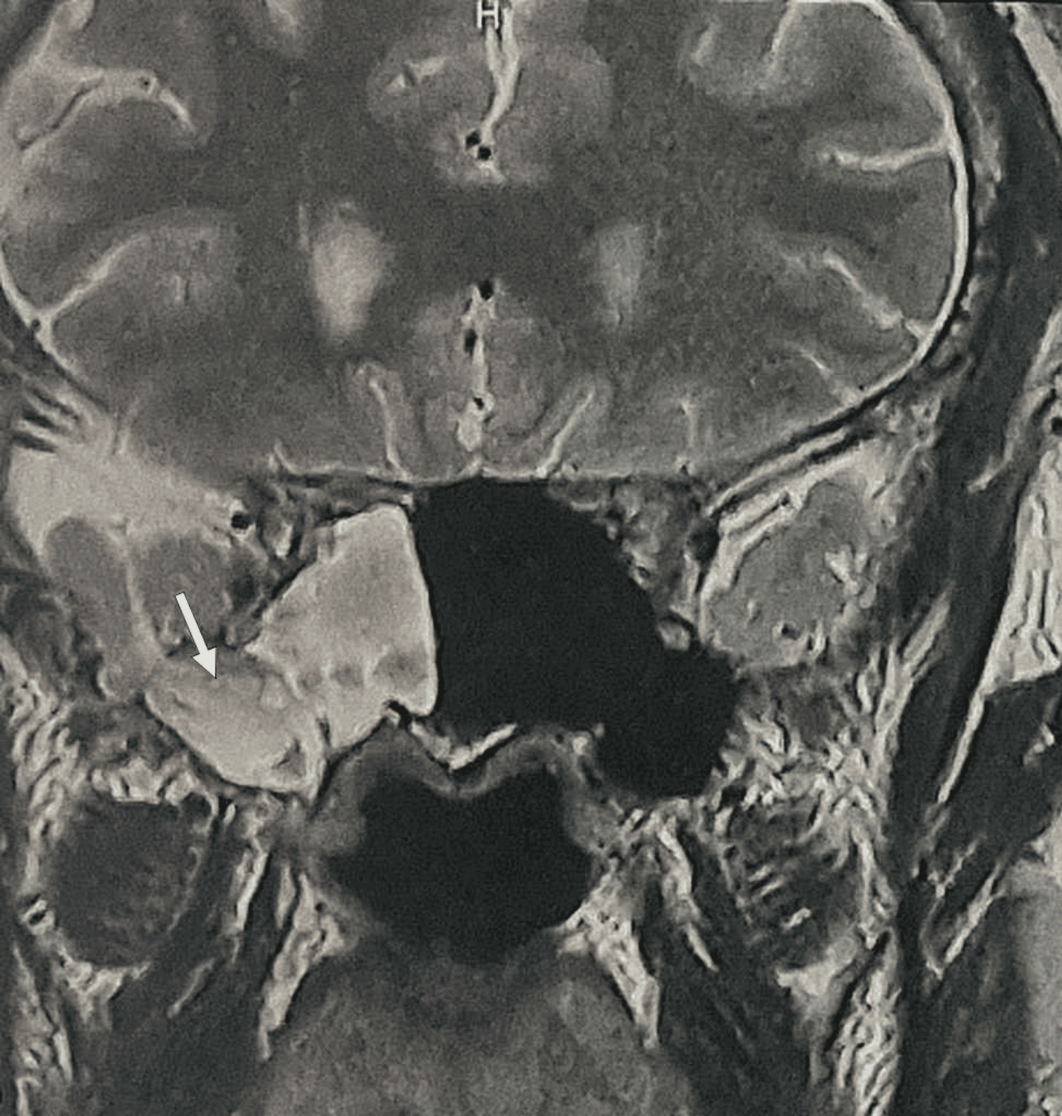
Coronal MRI demonstrating an osteomeningeal defect in the posterolateral wall of the sphenoid sinus associated with a meningoencephalocele (arrow) MRI: magnetic resonance imaging
